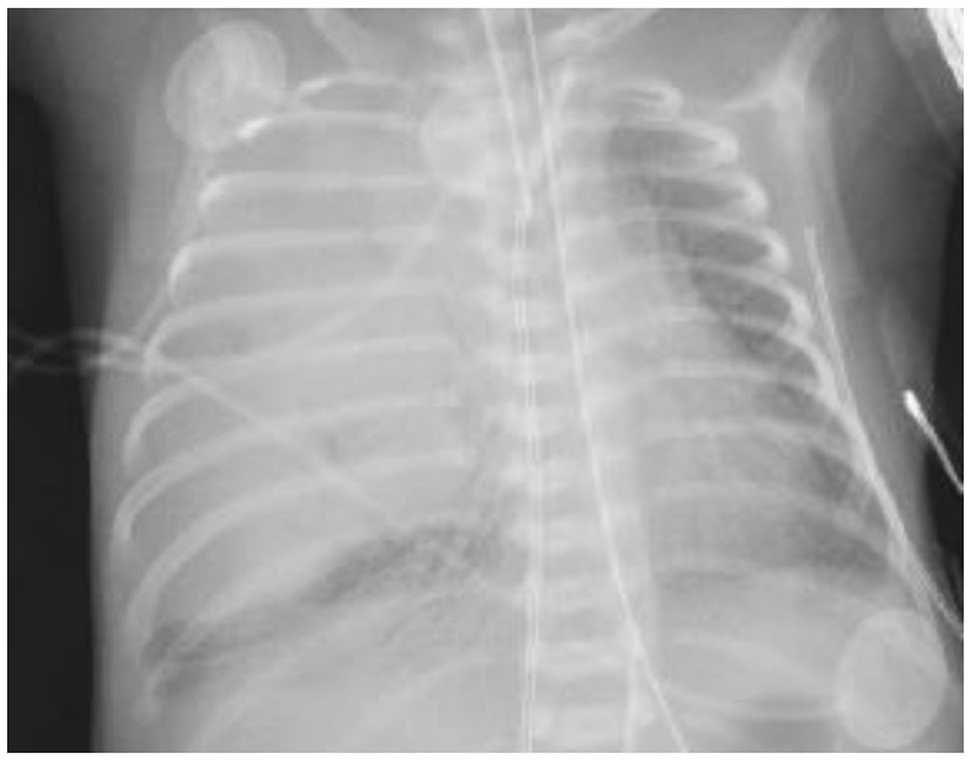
Anterior-Posterior Chest X-Ray at 5 h of Age, Right Lung Field Homogenous Opacification
